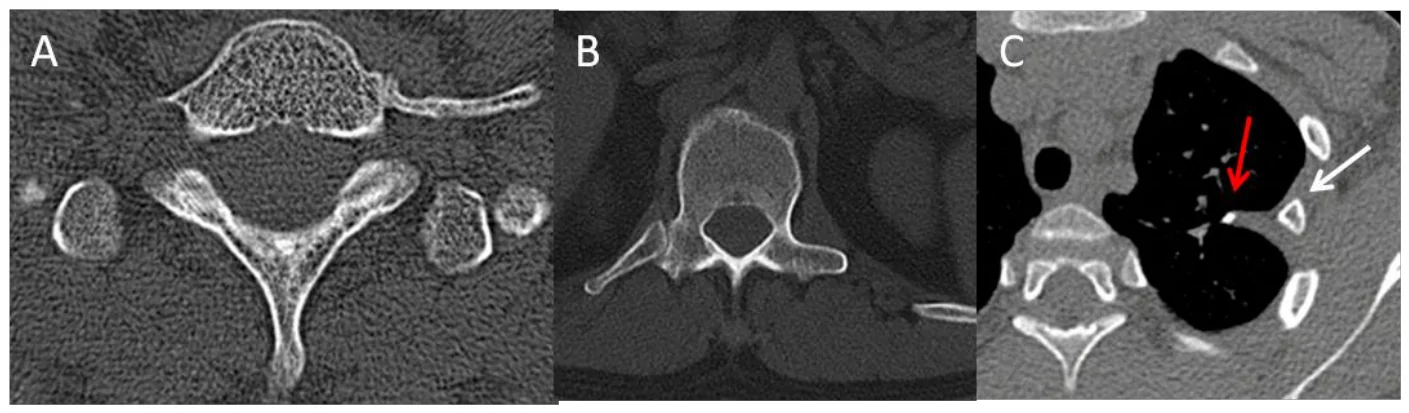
Axial CT images of accessory and supernumerary ribs. (A) A cervical rib. (B) A lumbar rib. (C) Thoracic CT demonstrating a supernumerary rib (red arrow) arising from the posterior aspect of a short left third rib (white arrow) and coursing inferolaterally toward the pulmonary parenchyma.
